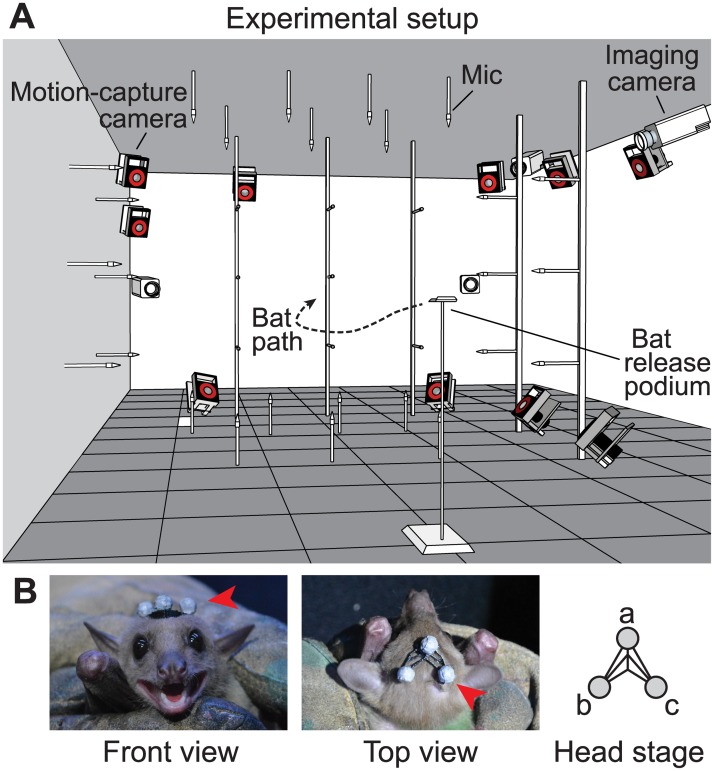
Setup of the experiment. (A) Experimental space and arrangement of instruments, including a three-dimensional array of 34 ultrasonic microphones, 10 high-speed motion-capture cameras, and 4 high-speed imaging cameras. (B) The head stage (indicated by red arrows) used to track the bat’s head movements.
